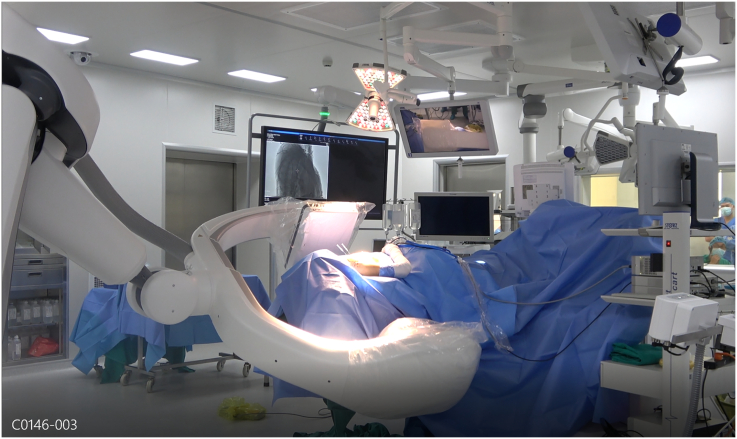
The robot used to perform the 3D CT scan.
